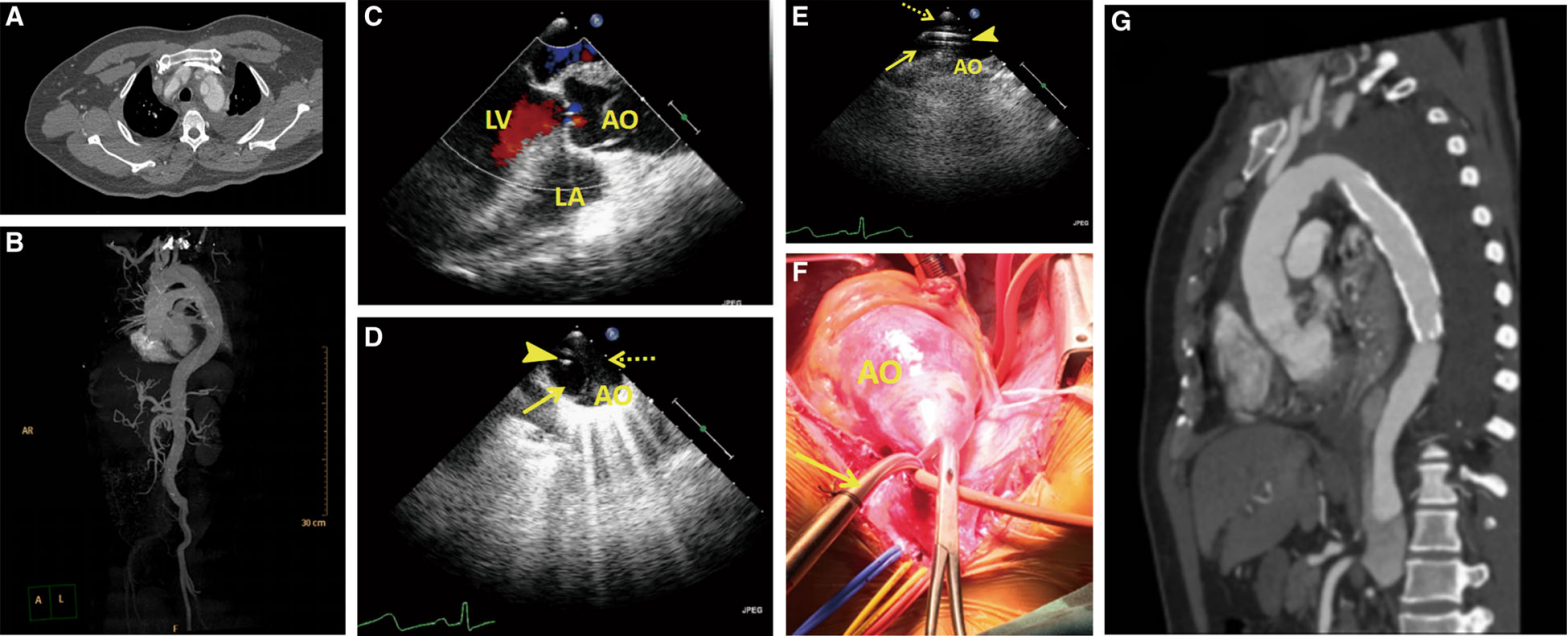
Perioperative images. a-b Computed tomography angiography (CTA) before the operation revealing a Stanford type A aortic dissection extending from the aortic root to the bilateral iliac artery. c Transesophageal echocardiography (TEE) showing a mild aortic regurgitation, an enlarged root (47) and ascending aorta (48–52), and an ejection fraction of 69%. d Transesophageal echocardiography (TEE) image. TEE showing the guide wire (arrow-head) present in the true lumen (arrow) of the descending aorta. The false lumen is depicted by a dotted-arrow. e Transesophageal echocardiography (TEE) image. TEE confirming the accurate positioning of the cannulation into the true lumen (arrow). Arrow-head is the false lumen. f The 20 Fr cannulation (Medtronic, arrow) advanced over the guide wire and cannulated into the true lumen of the aortic arch. g Postoperative CTA image revealing the patency of the three-branched vessels and the optimal position of the graft. Perioperative image. Computed tomography (CTA) before the operation revealing a Stanford type A aortic dissection extending from the aortic root to the bilateral iliac artery. Transthoracic echocardiography (TEE) image. Transthoracic echocardiography (TEE) showing a mild aortic regurgitation, an enlarged root (47) and ascending aorta (48–52), and an ejection fraction of 69%. Transesophageal echocardiography (TEE) image. TEE showing the guide wire (arrow-head) present in the true lumen(arrow) of the descending aorta. The false lumen is depicted by a dotted-arrow. Transthoracic echocardiography (TEE). TEE confirming the accurate positioning of the cannulation into the true lumen (arrow). Arrow-head is the false lumen. Transthoracic echocardiography (TEE). The 20 Fr cannulation (Medtronic, arrow) advanced over the guide wire and cannulated into the true lumen of the aortic arch. Postoperative CTA image. Postoperative CTA image revealing the patency of the three-branched vessels and the optimal position of the graft
